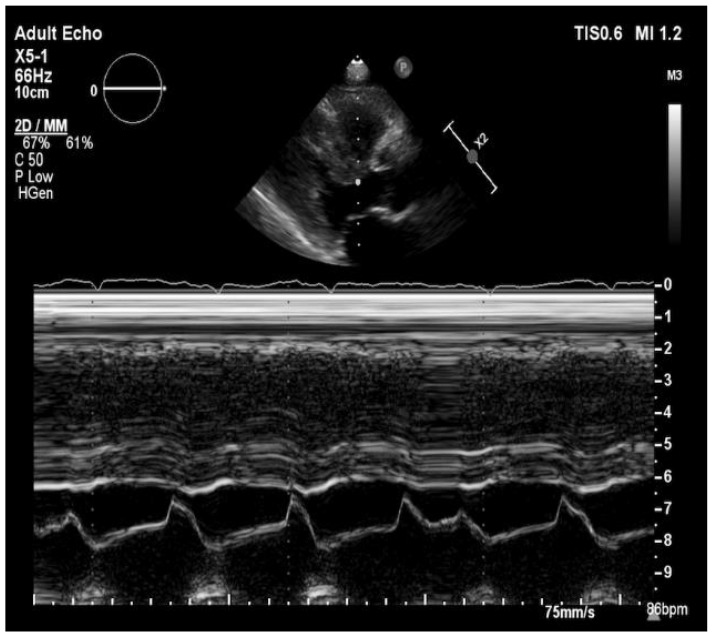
Transthoracic echocardiography in long parasternal view/M-Mode showing left ventricular hypertrophy.
